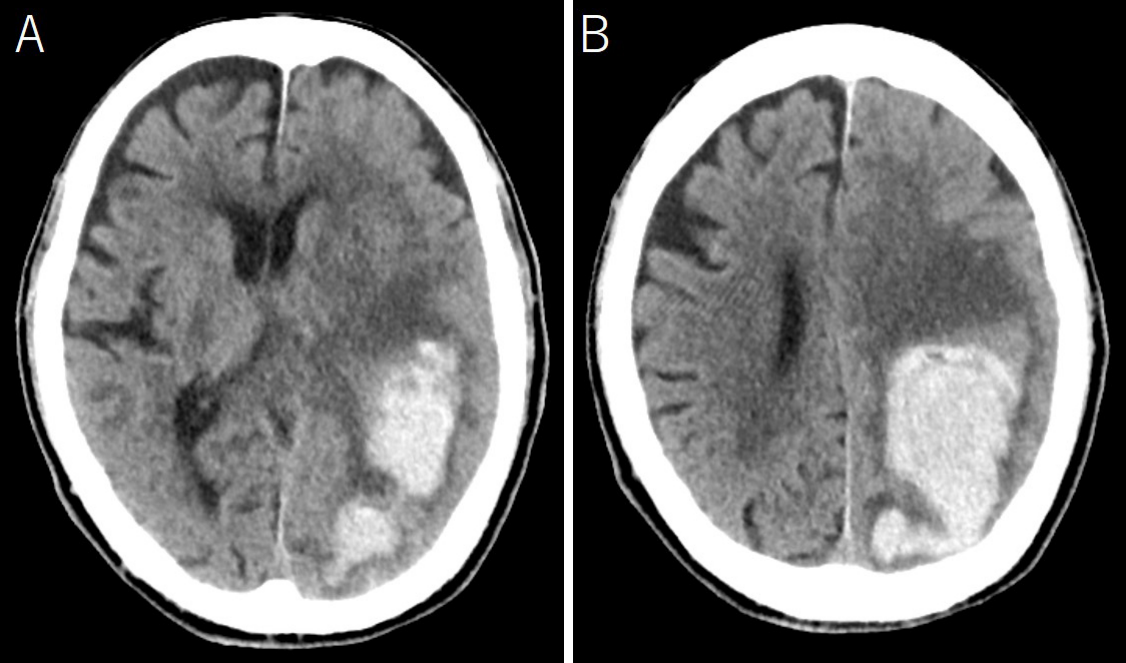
Computed tomography upon admission showed a large subcortical hematoma in the left parietal lobe.
